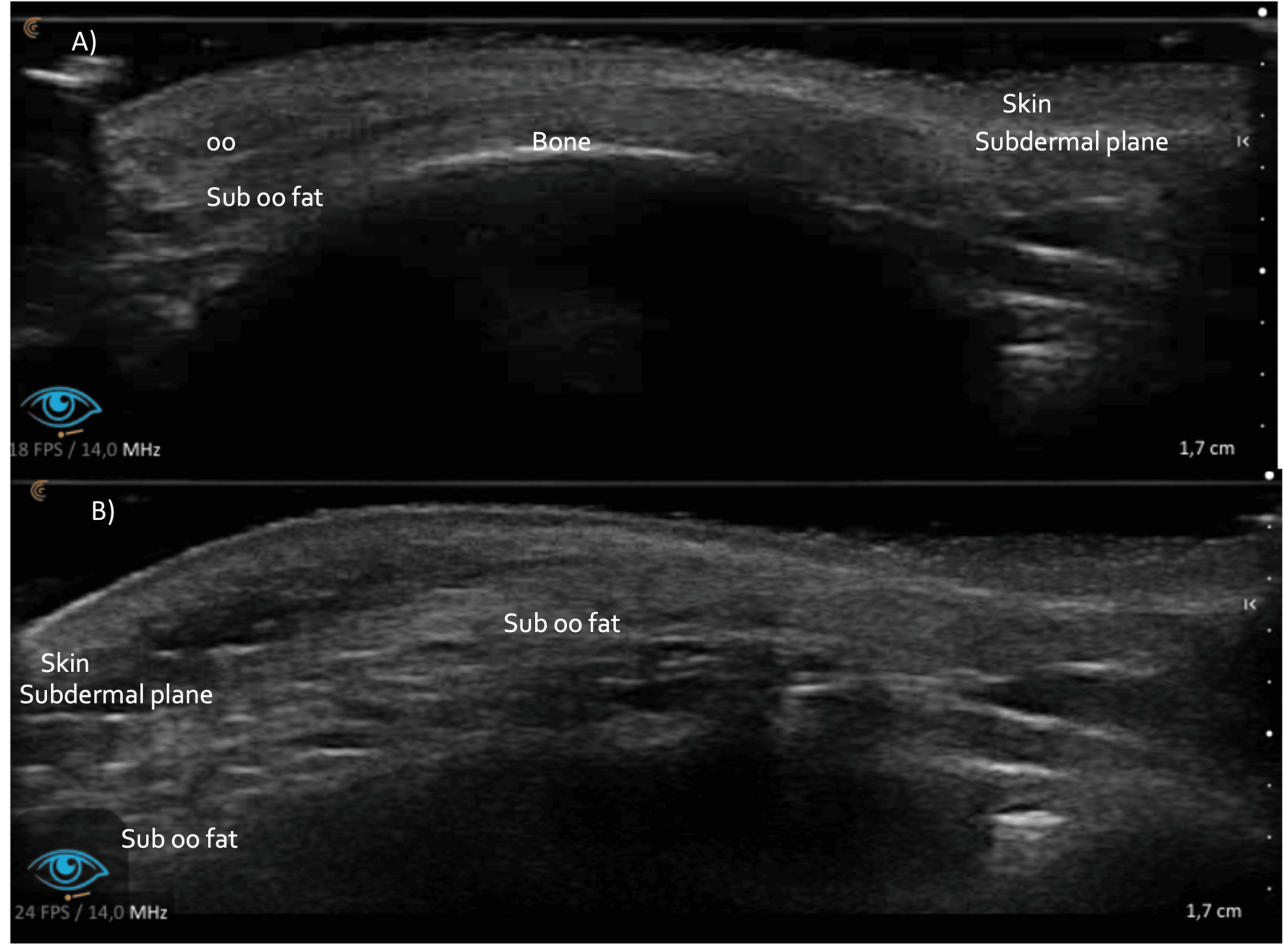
Skin and soft tissue high-resolution ultrasound two weeks post-treatment: Normal soft tissue ultrasound patterns. Images are shown using the medial-view (A) and lateral-view (B).
